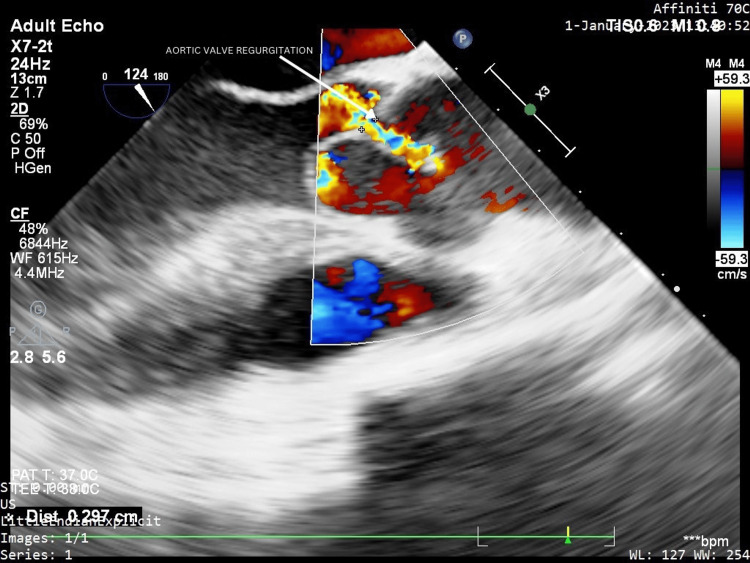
Aortic regurgitation visible on color Doppler transesophageal echocardiography. The above image has been taken by the authors.
